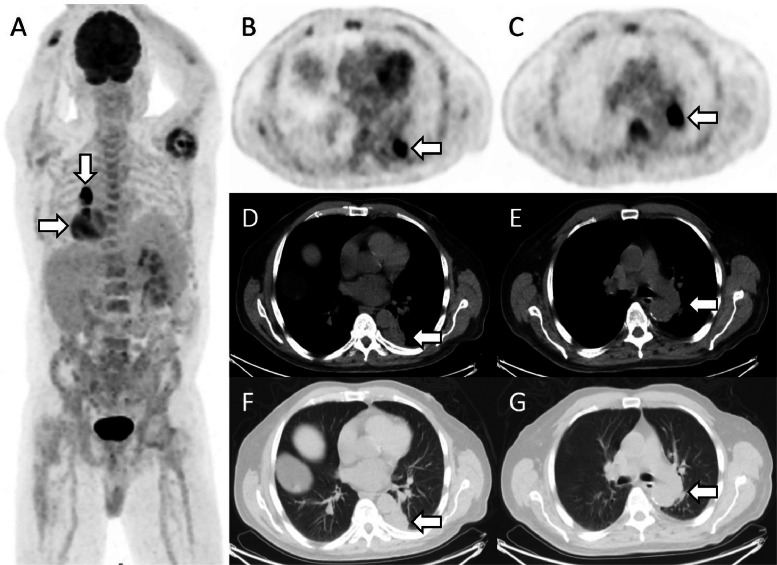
^18^F-FDG PET/CT maximal intensity projection image and CT images at presentation.
